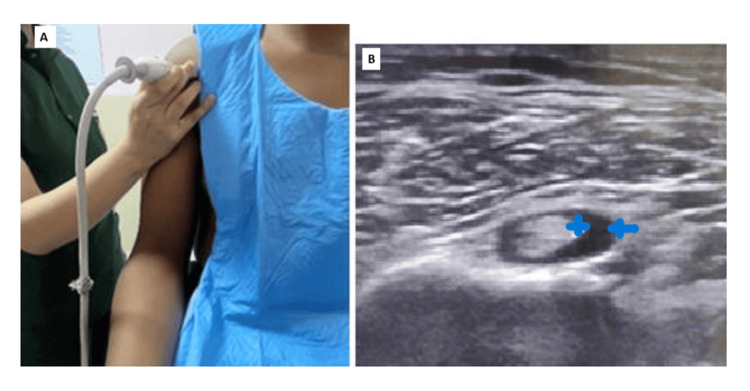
Measurement of long head of biceps tendon effusion by ultrasonography A) Position of the probe; B) Ultrasound image
